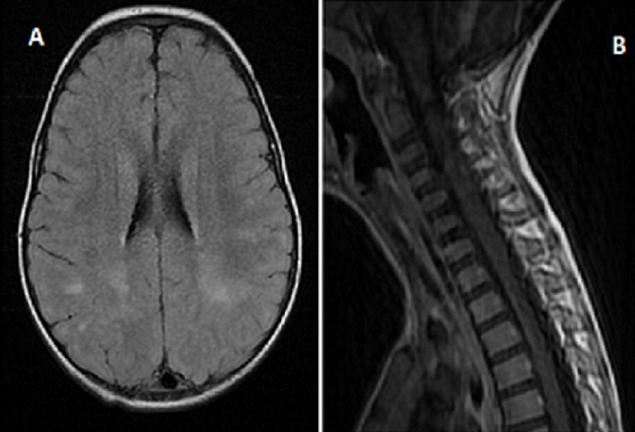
(A, B): IRM cérébro-médullaire séquence T2 FLAIR; lésions multifocales en hyper-signal
